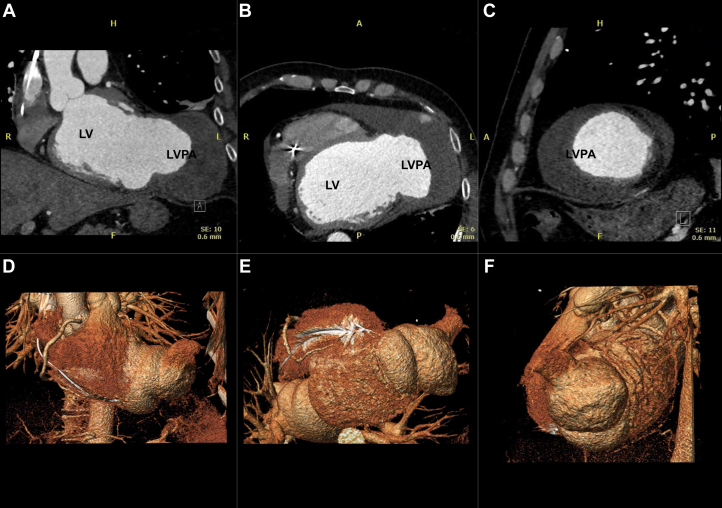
Dual-Source Cardiac Computed Tomography Angiography and 3-Dimensional Volume Rendering (A and D) Coronal views. (B and E) Axial views. (C and F) Sagittal views.
